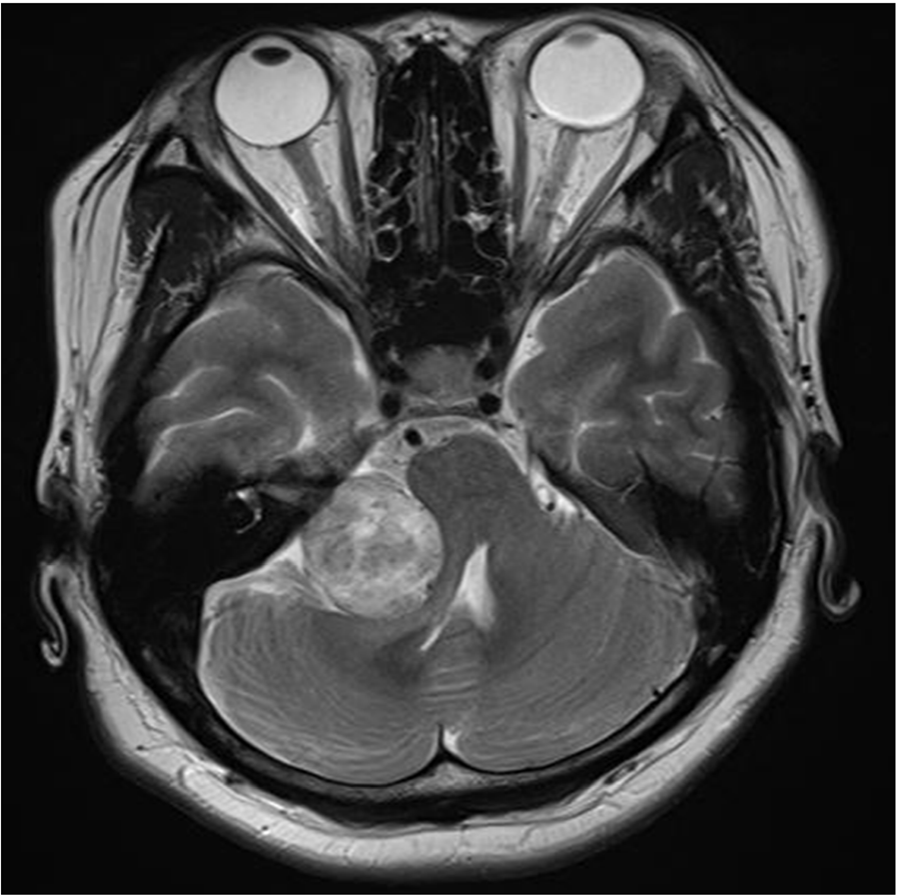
MRI image. Axial magnetic resonance image shows a 30 mm×30 mm well-defined mass involving the right cerebellopontine angle
